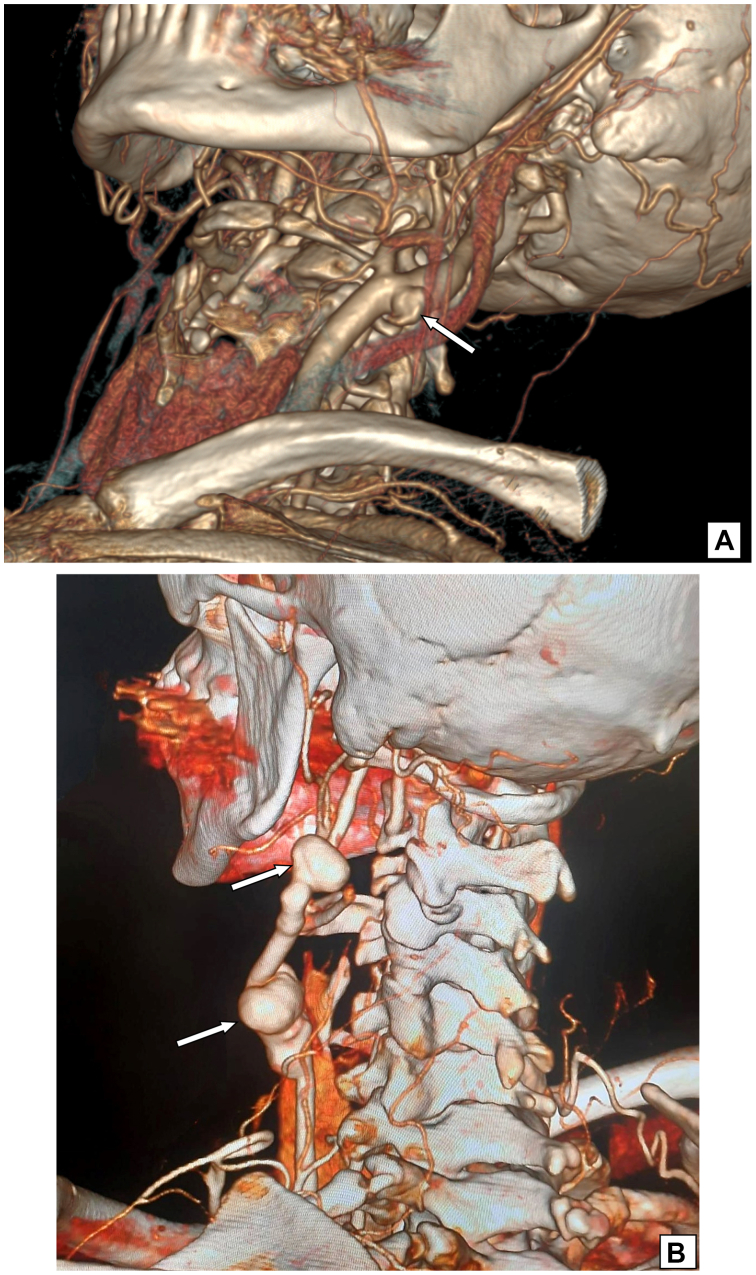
Computed tomography angiography of head and neck (three-dimensional reconstruction of neck vessels). (A) Pseudoaneurysm (PA) of left internal carotid artery due to giant cell arteritis; (B) PAs of the carotid vein graft anastomoses.
